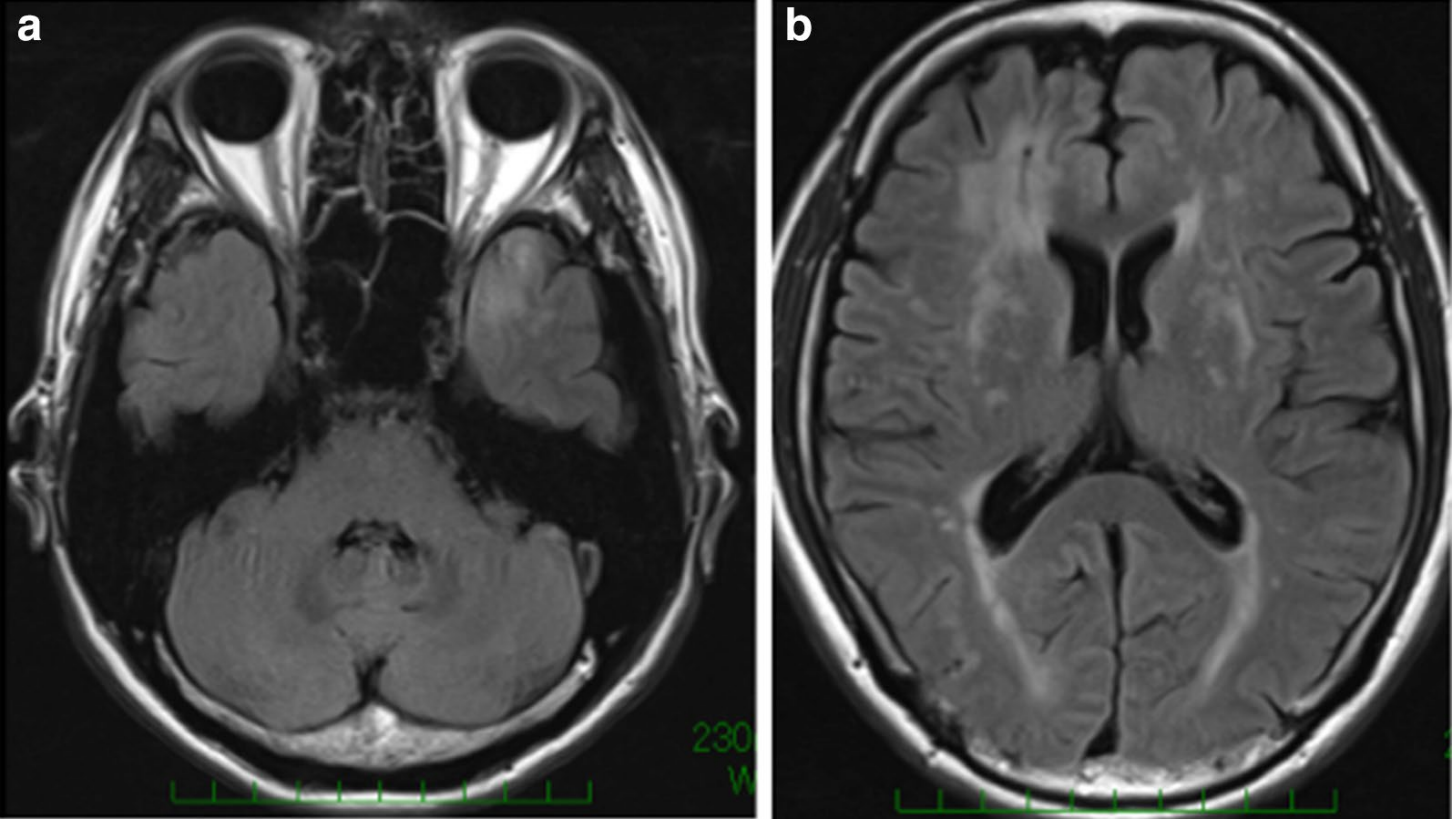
MR imaging (fluid-attenuated inversion recovery) demonstrated typical white matter increased T2-signal intensity abnormalities in the anterior temporal lobe (a) and external capsules (b)
